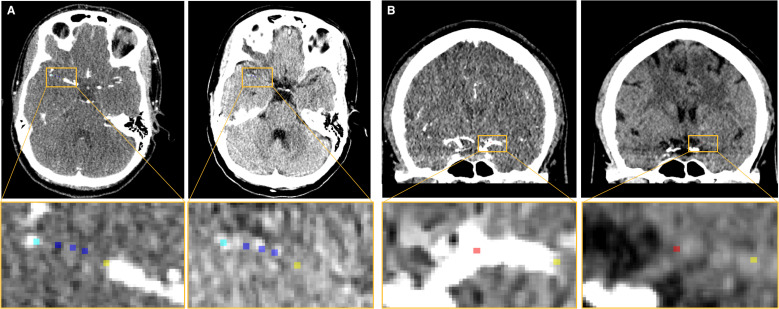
(A) CTA and NCCT scans of an AIS patient with a right MCA occlusion. Yellow and cyan markers indicate the proximal and distal border of the thrombus, respectively. The aim of the placement of the blue markers within the thrombus is twofold: aid in the computation of the thrombus length; and serve to create the spherical regions of interest for the thrombus attenuation and perviousness. (B) CTA and NCCT scans of an AIS patient with a left MCA occlusion. red and yellow markers indicate the location of the ICA-T and proximal thrombus border, respectively. AIS, acute ischemic stroke; CTA, CT angiography; ICA-T, terminus of intracranial carotid artery; MCA, middle cerebral artery; NCCT, non-contrast CT.
